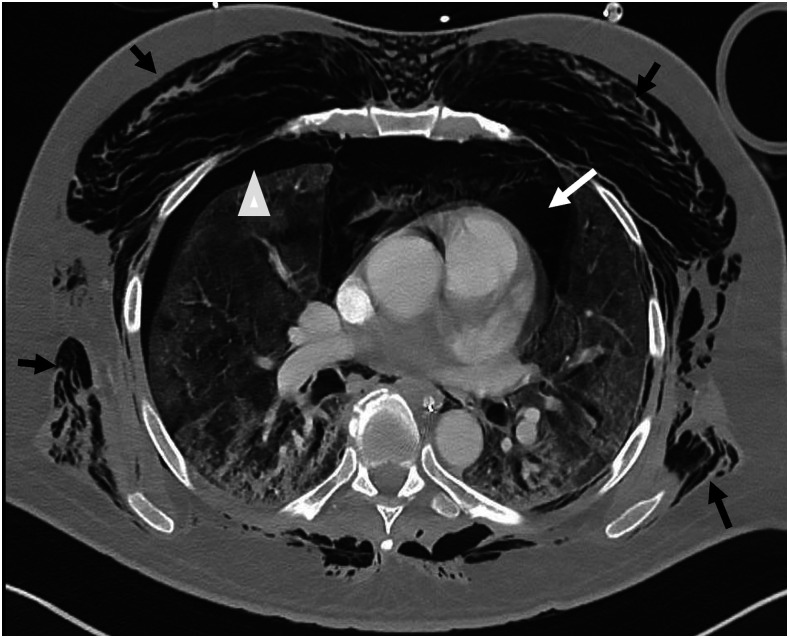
Computed tomography (CT) examination of the chest showing extensive surgical emphysema (black arrows), pneumomediastinum (white arrows), and bilateral pneumothorax (arrowhead).
